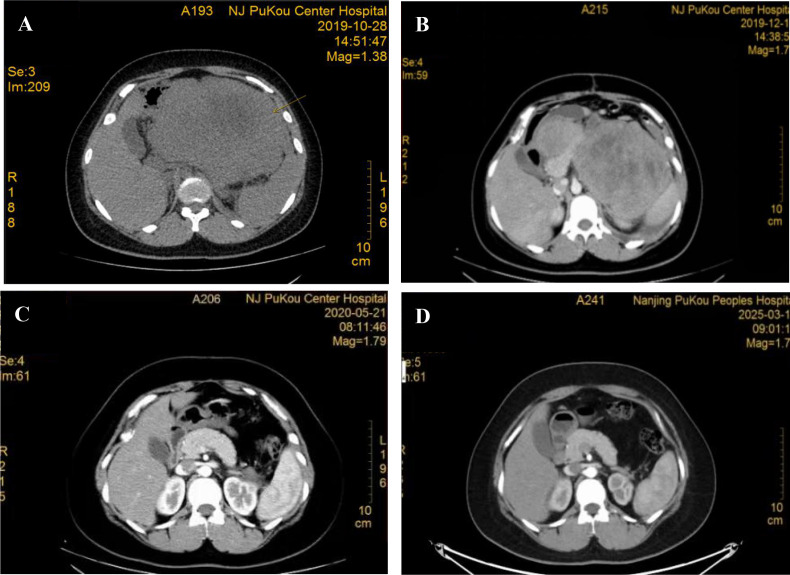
Changes of radiography at different period of treatment. (A) CT scan performed at baseline (October 28, 2019); (B) CT scan performed at one month after surgery when early progression with new metastases (December 13, 2019); (C) CT scan assessment of best response of CR (May 21, 2020); (D) Recent CT scan assessment of CR (March 11, 2025);.
